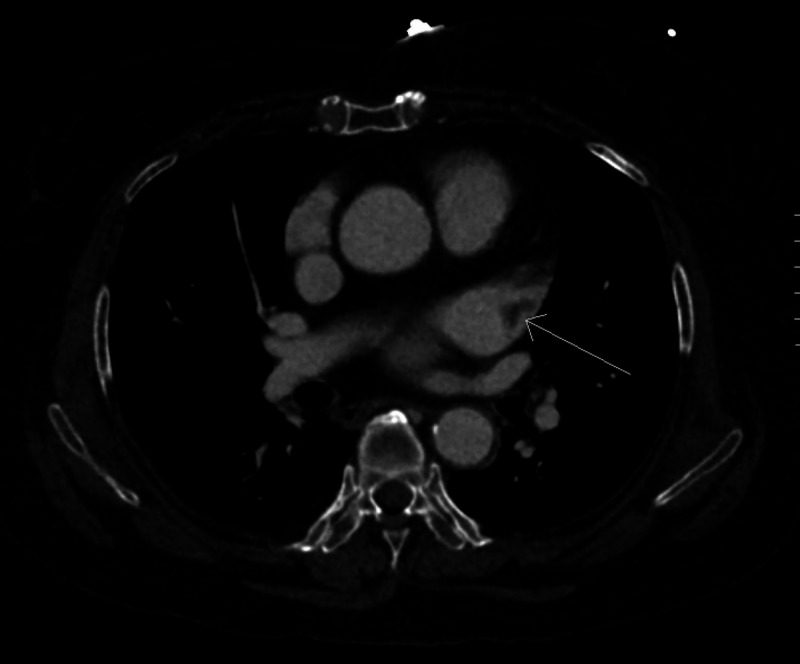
Coronal CT angiography demonstrating 1.8-cm left atrial appendage thrombus (arrow)
